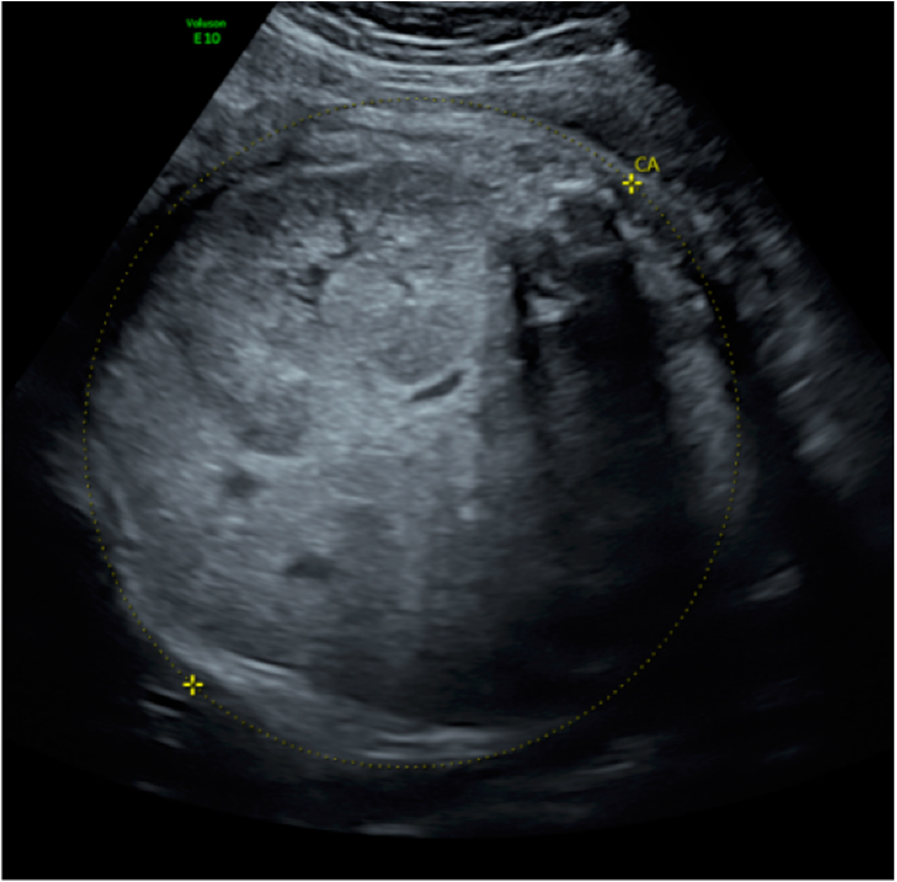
Ultrasound at 35 weeks of gestation with abdominal circumference measurement
